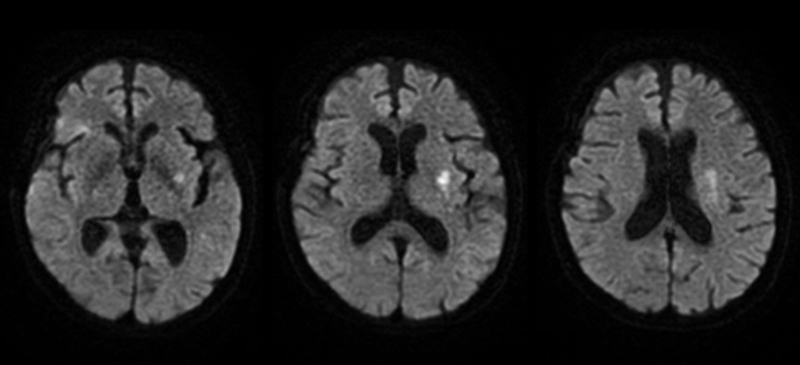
Brain MRI. Diffusion-weighted brain MRI showed ischemic stroke located between the posterior limb of the left internal capsule and the putamen. MRI: magnetic resonance imaging.
